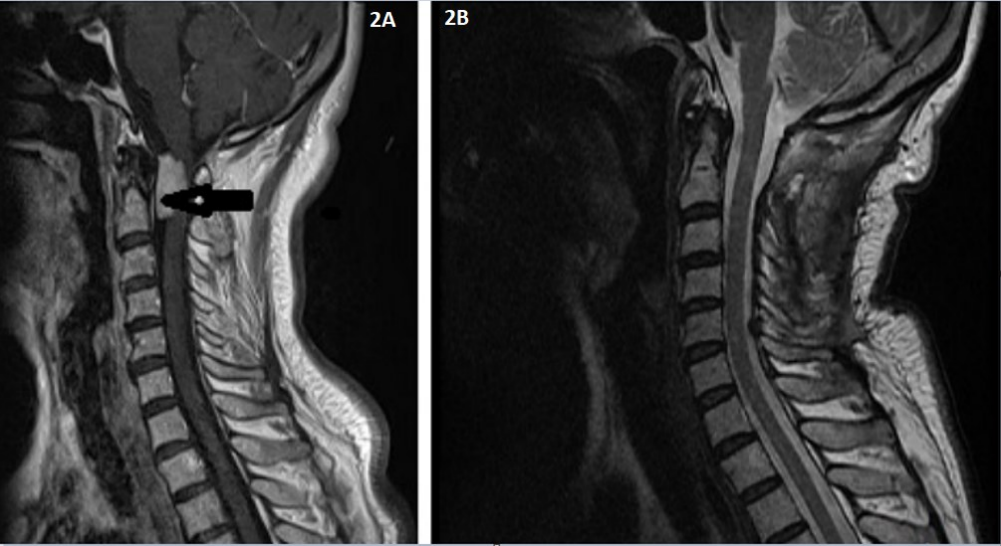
68 years old female patient (A) pre-operative sagittal MRI, (B) post-operative sagittal MRI. Black arrow indicates tumor
